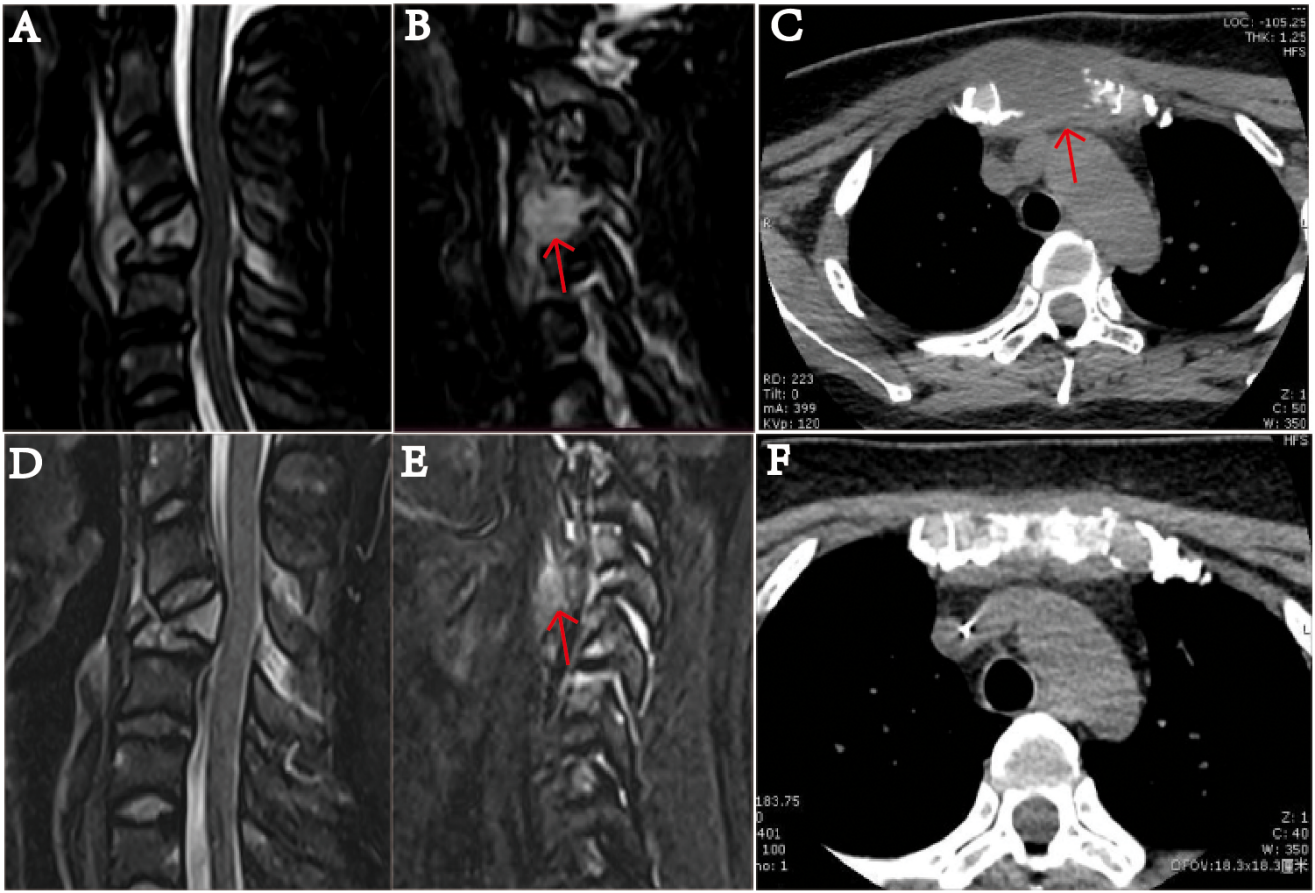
MRI examination of the cervical vertebra of the patient on January 20, 2022. (A) shows slope, C4, T1 vertebrae, and ancillary multiple bony lesions with pathologic fracture of the C4 vertebrae. (B) shows a 1.49 mm ×1.54 mm mass (indicated by the arrow) near the C4 cone, and the C4 vertebral body is compressed and flattened and shows pathological fracture. (C) The sternum is fractured, and there is a 37.41 mm × 31.14 mm soft tissue mass (indicated by the arrow). On March 19, after receiving two courses of chemotherapy, the patient showed destruction of multiple bones and a cervical spine fracture on MRI (D–F). In part (E), the C4 paravertebral mass is significantly reduced (indicated by the arrow). In part (F), the soft tissue mass causing the sternum bone fracture is essentially undetectable.
